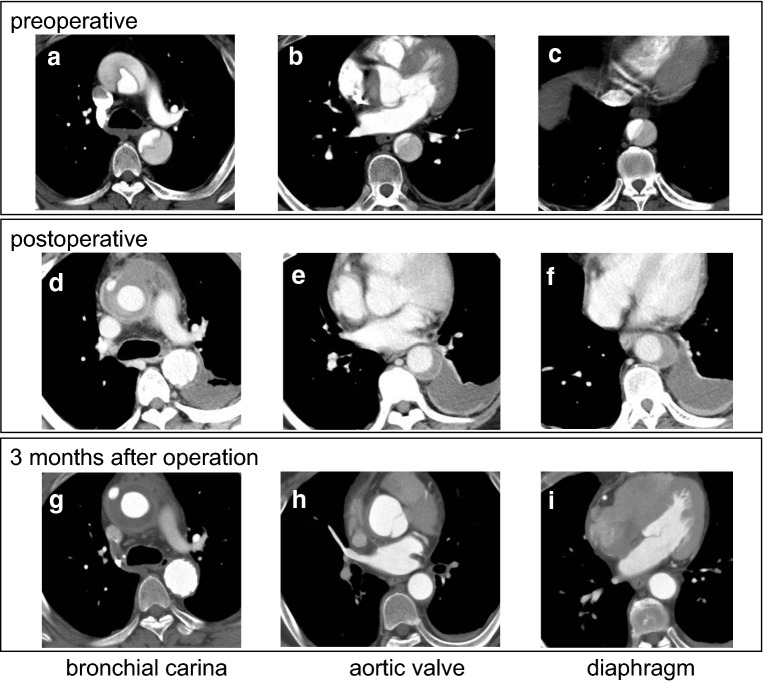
CT scan of a patient treated with TAR with FET. Preoperative CT image (a–c), postoperative image before discharge from the hospital (d–f), and at 3 months after the operation (g–i) at the level of bronchial carina (a, d, g), aortic valve (b, e, h), and diaphragm (c, f, i), respectively. Note excellent aortic remodeling of downstream aorta after TAR with FET with the complete thrombosis of false lumen and expanded true lumen at each level
